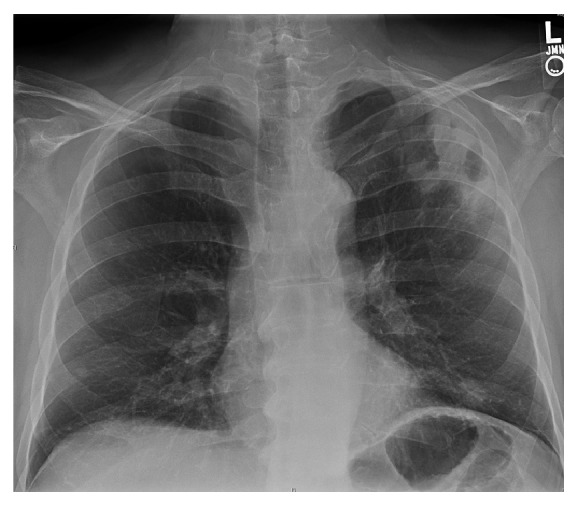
Chest X-ray at day of admission.
